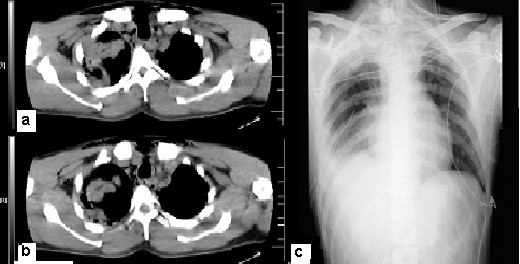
Computed tomography scan (a,b) and the anteroposterior view X-ray (c) of the chest after cervical surgery. It revealed a pneumothorax, much fluid, and pulmonary atelectasis on the right side of the chest with pneumomediastinum.
